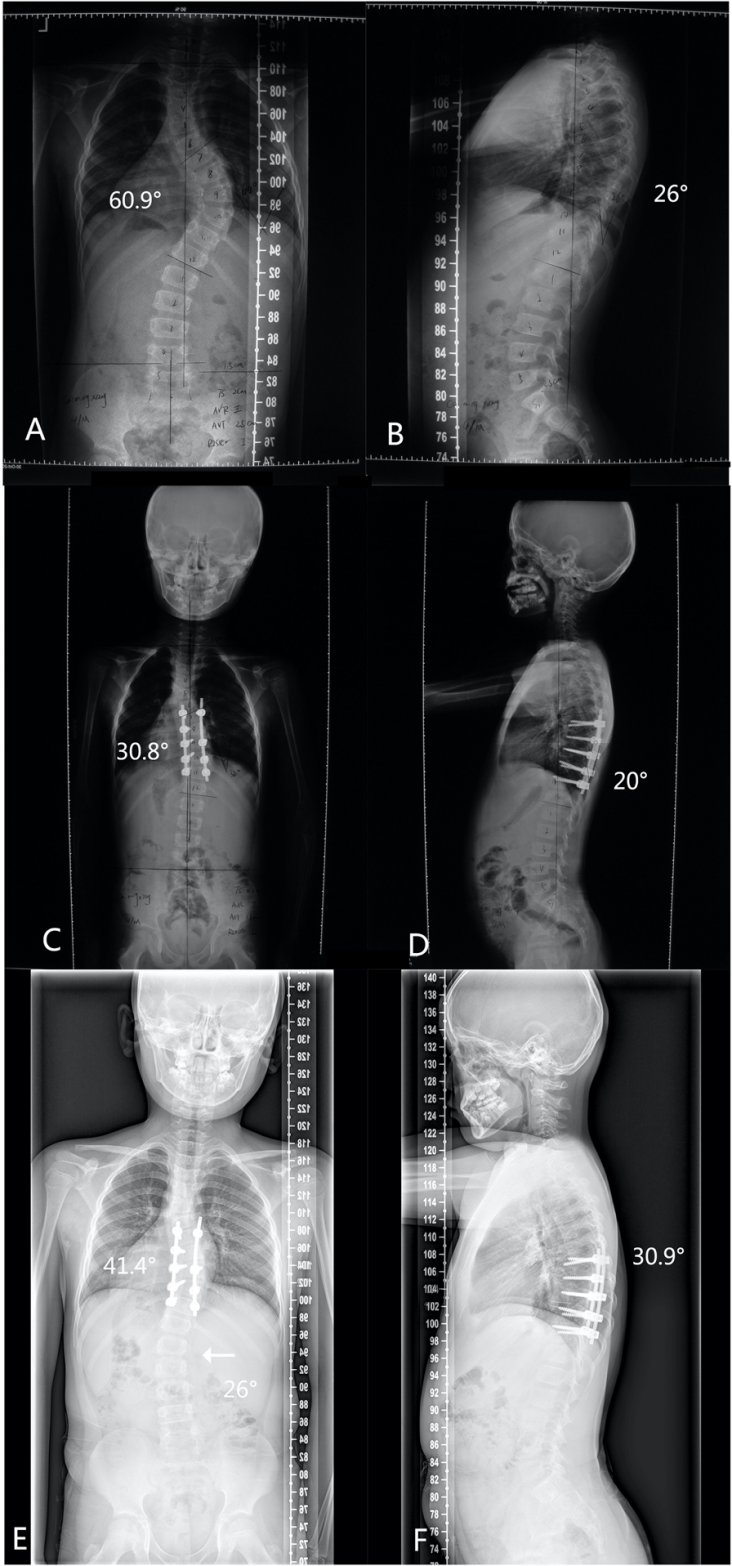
Case 6: Radiographs of a 4-year-old patient with neurofibromatosis and a 60.9°right thoracic scoliosis, who received posterior only fusion operation from T7-T11. A.B. Preoperation. C.D. Postoperation. E.F. The adding on phenomenon (arrow) was obvious at the 34-month follow-up, which was attributed to at the growth of the anterior column of the fusion segments
